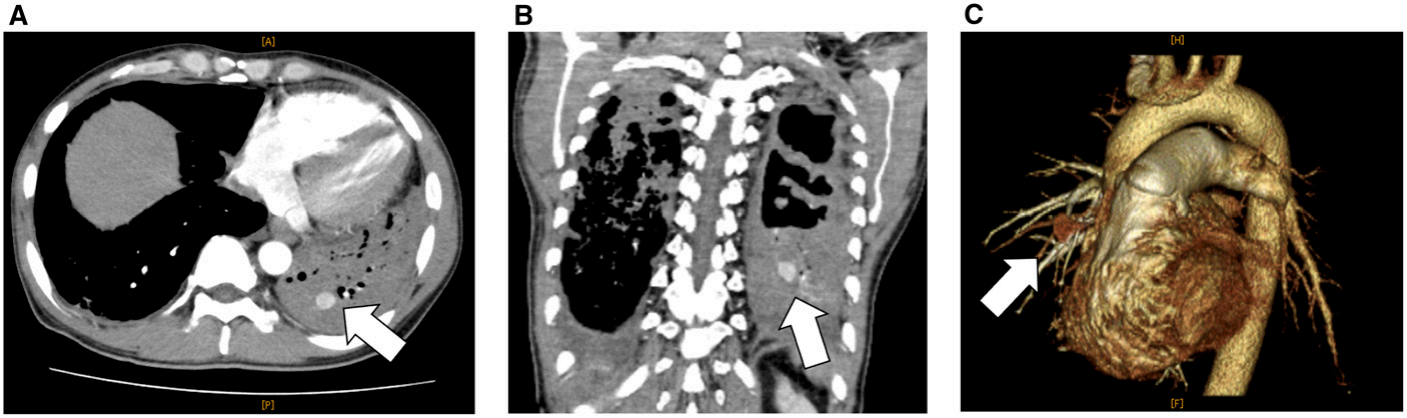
Axial view (A) and coronal view (B) of contrast-enhanced computed tomography (CT) and CT angiography with three-dimensional reconstruction (C) showing an 11-mm-diameter pseudoaneurysm in the left lower pulmonary artery (white arrow) surrounded by high-density fluid collection. This figure appears in color at www.ajtmh.org.
